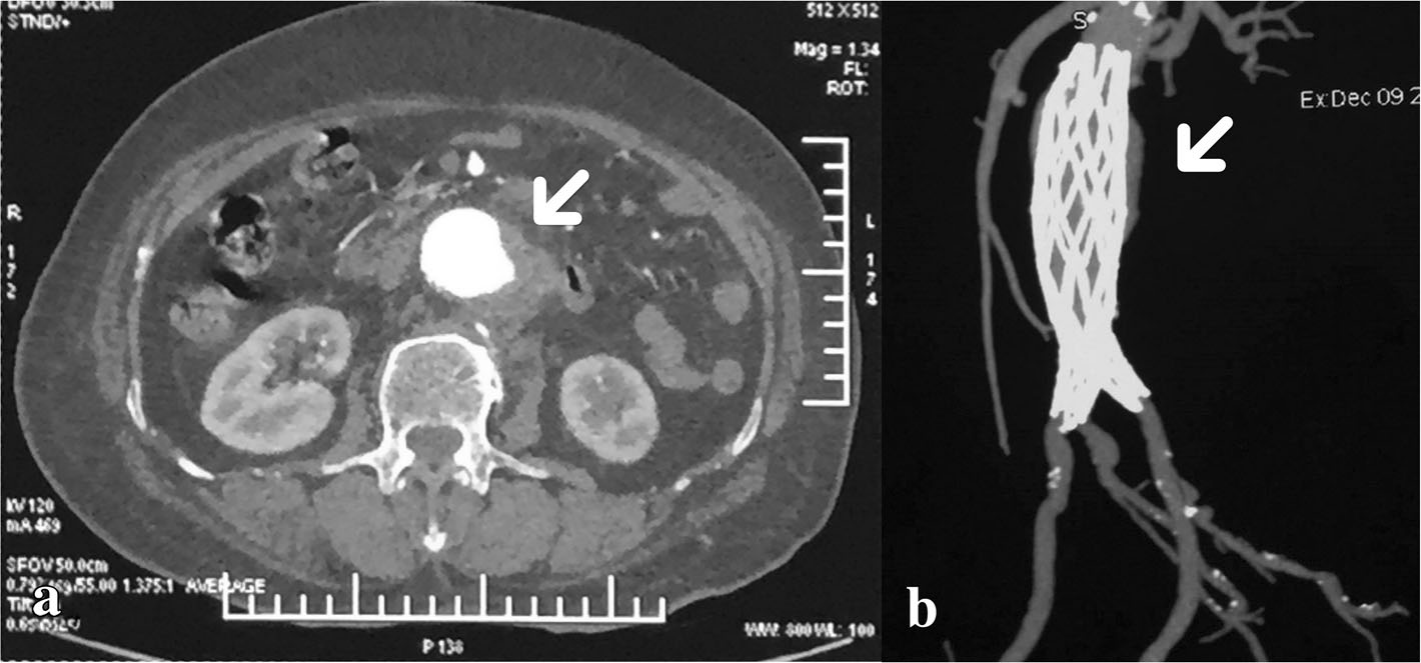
CT was taken before endovascular repair. The contrast medium spilled through the fabric tear in the left wall of middle segment of the stent (arrows both in a and b)
